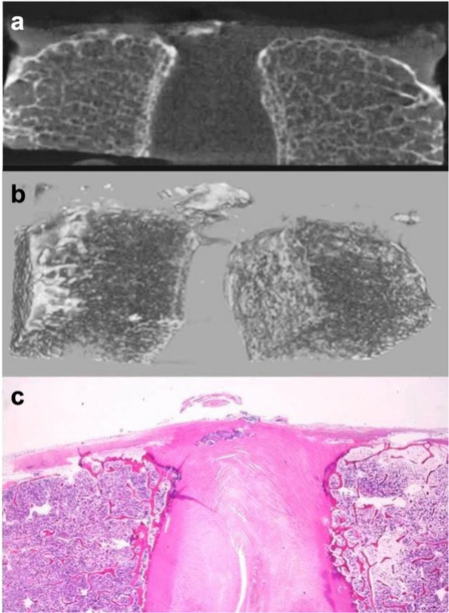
a–c Representative case of the isolated posterior-annular fibrosus type. a CT image: The high-density area was interpreted as a collection of small lesions instead of a single high-density area. b Three dimensional reconstruction image: The high-density area was present at isolated intervertebral levels and had no continuity to the vertebra. c Pathological image: These cases typically showed the prolapse of degenerated nucleus pulposus with previous rupturing of annular fibrosus of the intervertebral disc, accompanied by fibrosis, calcification and/or ossification
